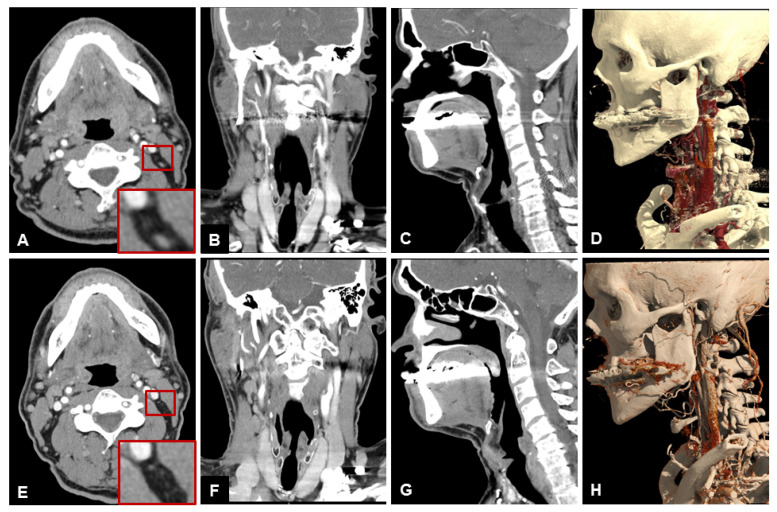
Example of a 57-year-old male patient with a squamous cell carcinoma of the lateral border of the tongue. Patient underwent initial resection. Both NR-CT and UHR-CT were performed after resection, and the carcinoma shows no signs of recurrence. Note the remarkably improved delineation of muscle and vessels with UHR-CT. (A) axial 3 mm reconstruction NR-CT, (B) coronal 3 mm reconstruction NR-CT, (C) sagittal 3 mm reconstruction NR-CT, (D) best possible 3D-reconstruction NR-CT, (E) axial 3 mm reconstruction UHR-CT, (F) coronal 3 mm reconstruction UHR-CT, (G) sagittal 3 mm reconstruction UHR-CT, (H) best possible 3D-reconstruction UHR-CT.
